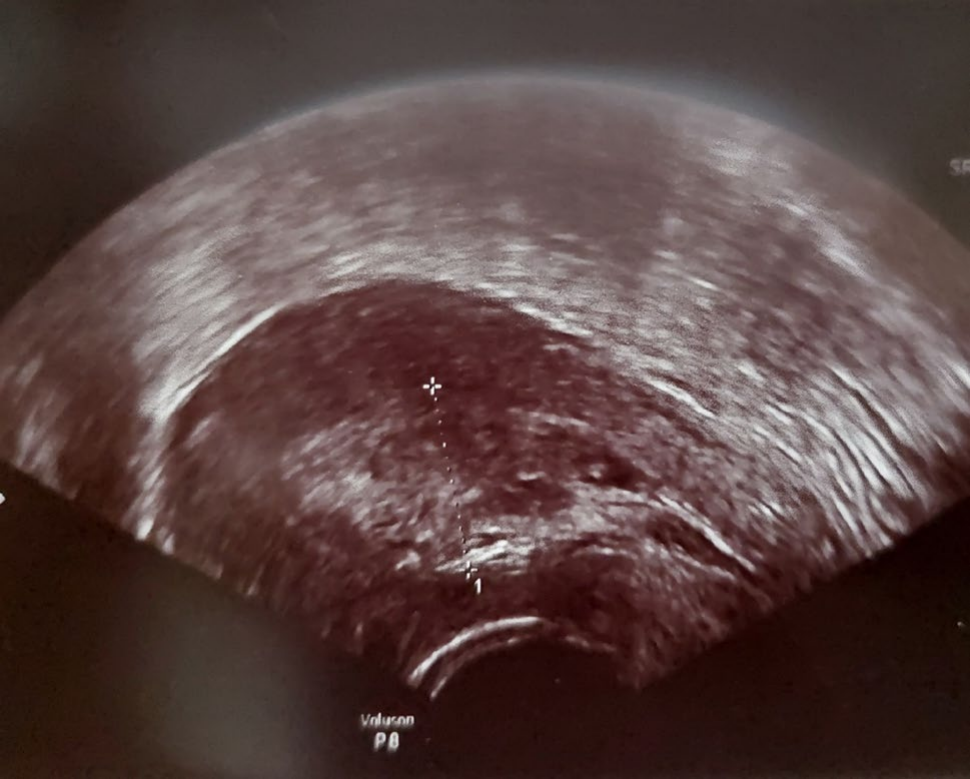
Endometrial stripe thickness per ultrasound
